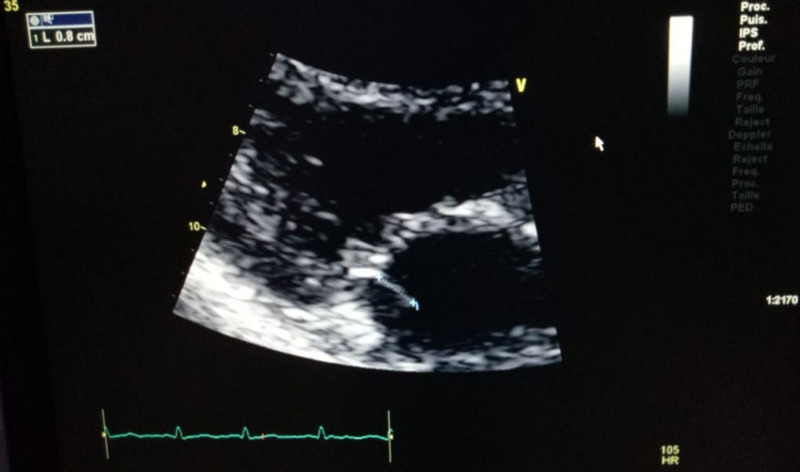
Transthoracic echocardiography showed 0.8 × 0.2 cm vegetation on the patient’s mitral valve in the parasternal long-axis view
